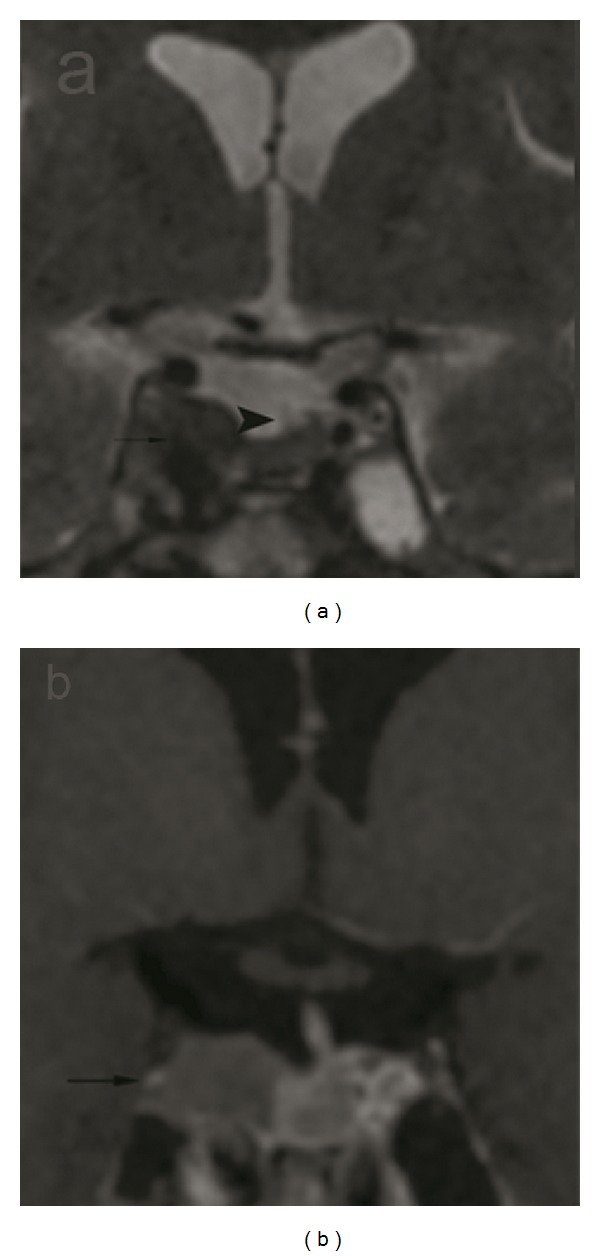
A hyperintense macroadenoma with the infindibulary deviation (arrow heads) and the invasion of the right cavernous sinus (arrows) are shown on the T2-weighted coronal image (a) and T1-weighted coronal image enhanced with IV-GBCM (b) of a patient.
